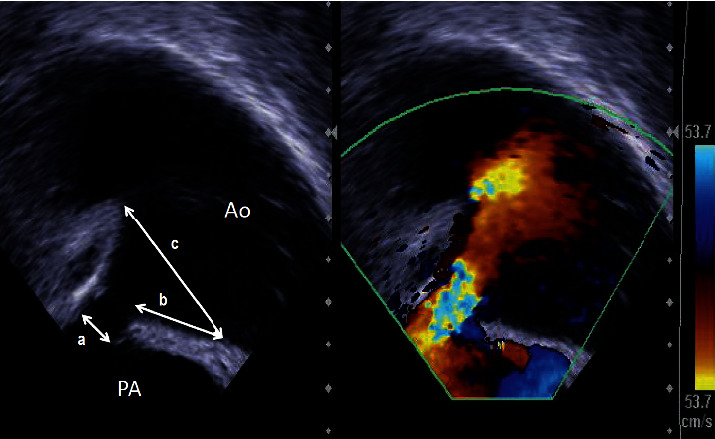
PDA measurement methods in LPA view using ICE. (A) The pulmonary artery side diameter of the PDA. (B) The lower contour PDA length between the end of the PA and the starting point of the aortic ampulla. (C) The aortic side diameter of the PDA. Ao, aorta; ICE, intracardiac echocardiography; PA, pulmonary artery; PDA, patent ductus arteriosus; LPA, left pulmonary artery.
